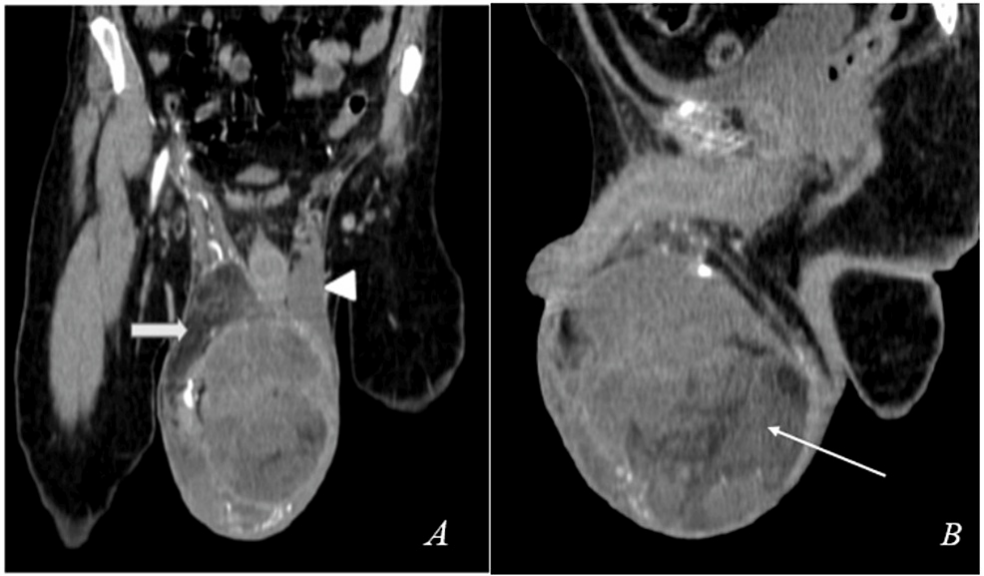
CECT abdomen coronal section (A) and sagittal section (B) showing a large mild heterogeneously enhancing mixed attenuating extratesticular lesion measuring approximately 12.7 x 10.9 x 9.3 cm with internal soft tissue and fat attenuating areas and a few peripheral punctate & chalky calcifications seen involving and expanding the right hemi-scrotum with lipomatous part of the tumour of right spermatic cord (white arrow in image A, white arrow in image B). The lesion is seen causing inferior and peripheral displacement of the right testis and significant compression and cranial displacement of the left hemi-scrotum and testis (white arrow head). CECT - Contrast-enhanced computed tomography
